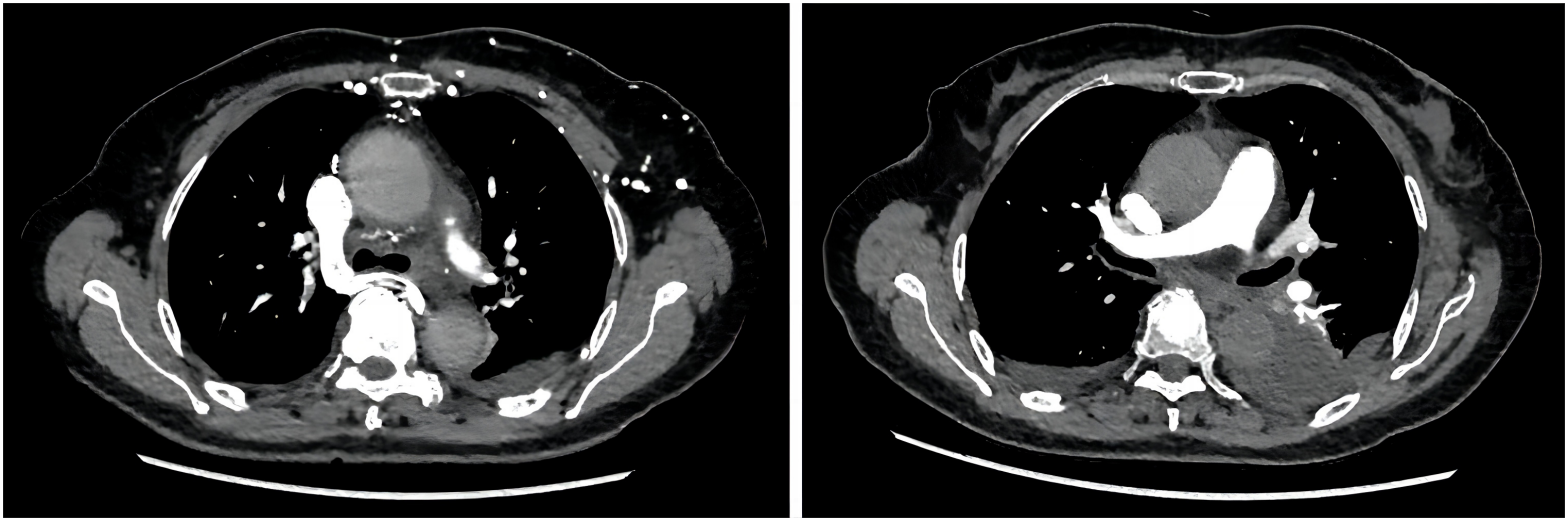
The computed tomography angiography examination revealed plaque-filled defects in the branches of the pulmonary artery.
